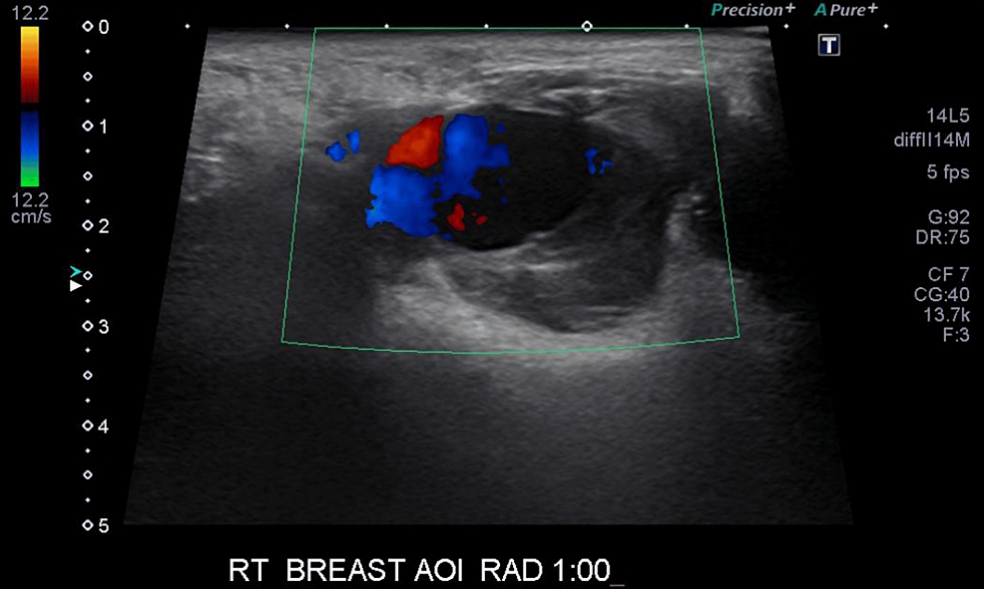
A single-spot image of gray-scale ultrasound with color Doppler analysis demonstrates bidirectional blood flow, or the Yin-Yang sign, consistent with a pseudoaneurysm
